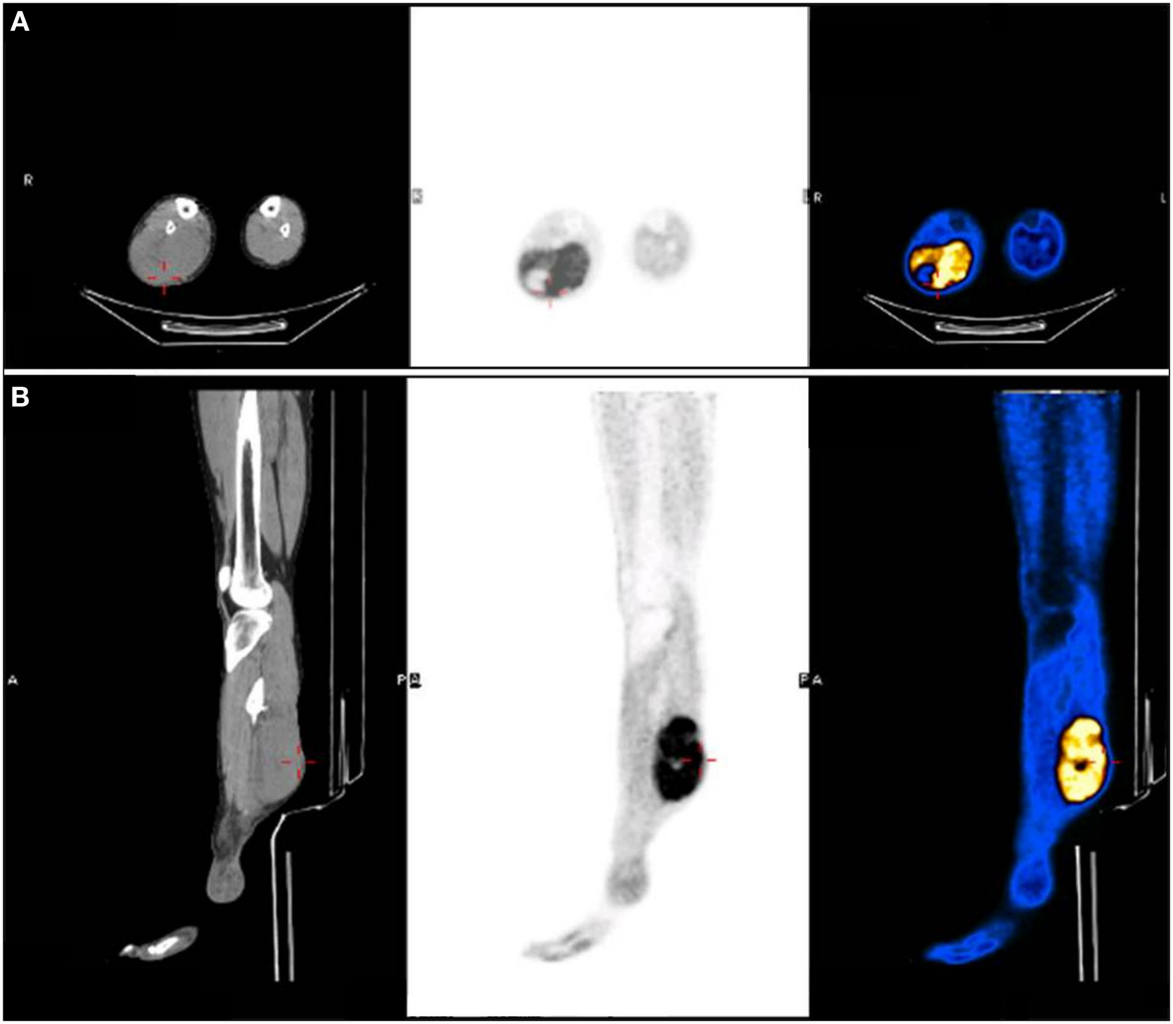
FDG-PET-CT indicated a hypermetabolic mass in the right calf. (A) Axial view. (B) Sagittal view.
